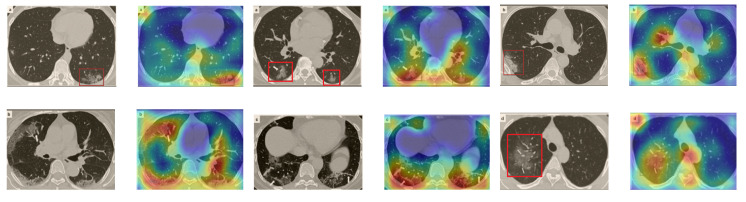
Grad-CAM visualizations for the same CT images in the first two rows of Figure 15. The CT images were correctly identified by SqueezeNet as COVID-19 cases with relevant localization of the disease-related regions.
